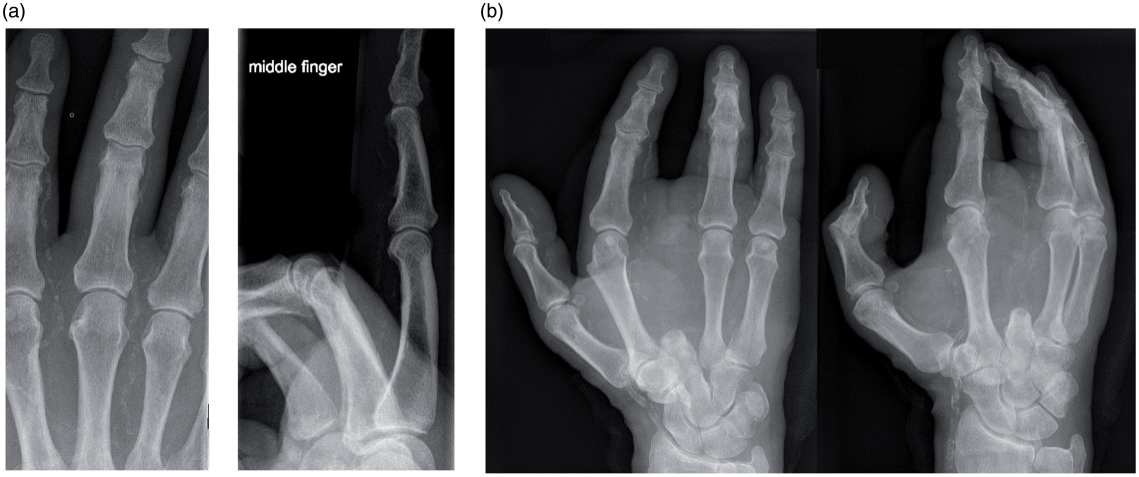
(a) Posteroanterior and lateral X-rays of a right middle finger from a 72-year-old man with arterial calcification taken at the time of diagnosis of osteomyelitis. (b) Posteroanterior and oblique X-rays taken 5 months later following phalanx amputation of the right middle finger at the proximal interphalangeal joint 1 month after diagnosis and then complete ray amputation a month later.
